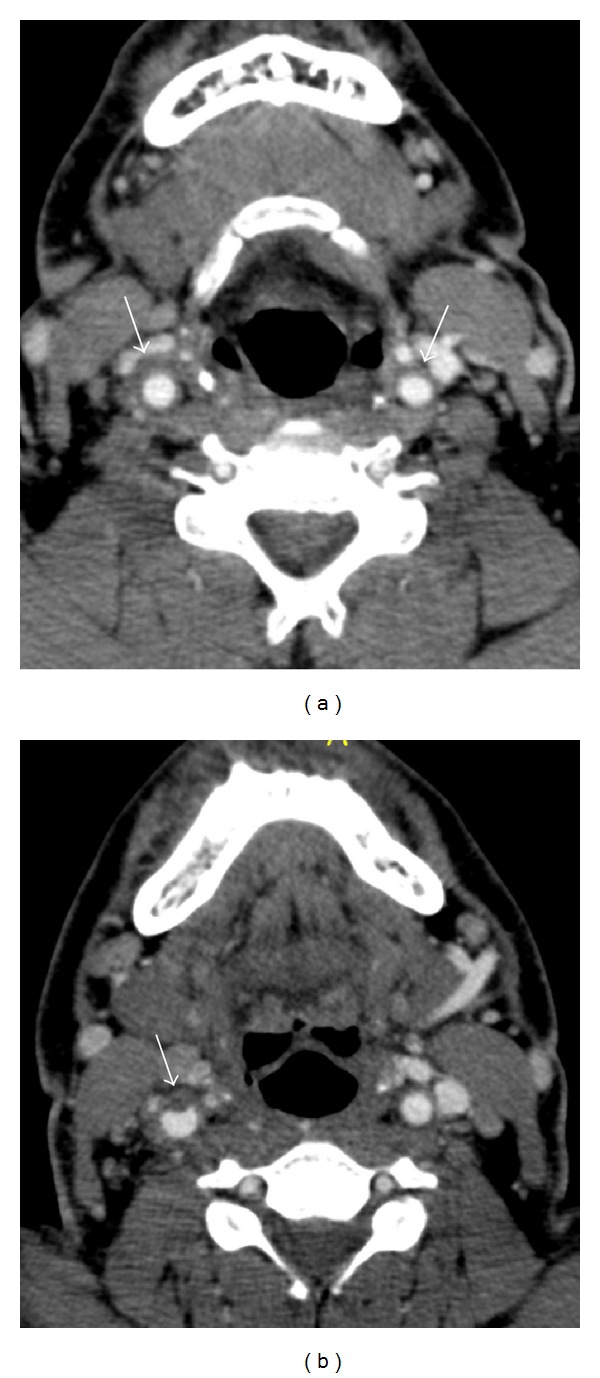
Axial CT angiography sections show wall thickening in both common carotid arteries (arrows, (a)) and a small thrombus protruding into the lumen of the right common carotid artery (arrow, (b)).
